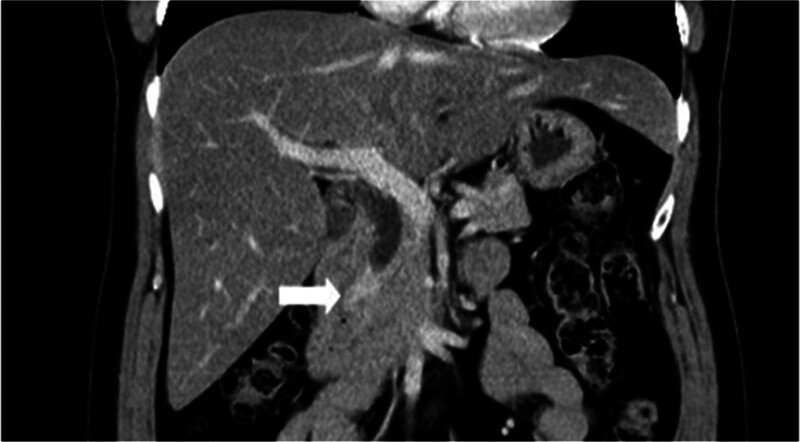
CT showed enhanced thick wall and tumor-like mass about 1.6 cm over distal common bile duct (arrow). CT = computed tomography.
